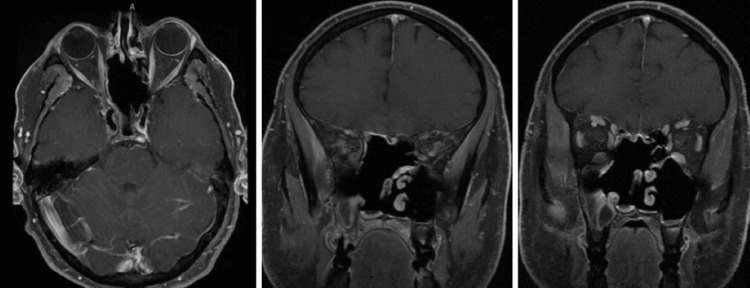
Eleven months post-treatment T1 fat-suppressed post-contrast MRI brain/orbit axial (A) and coronal planes (B, C). Extensive postsurgical changes with no definite signs of residual or recurrent tumor were noted.
